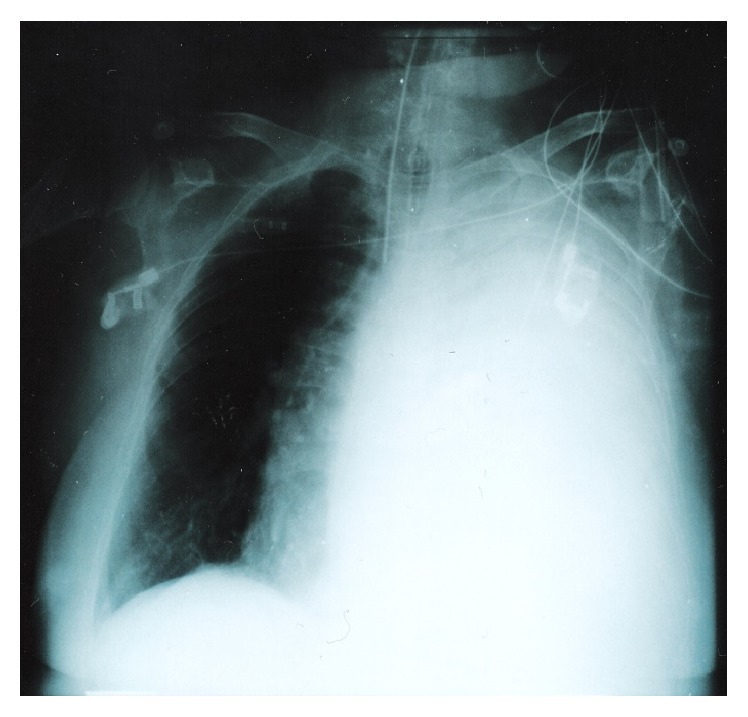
The admission chest X-ray showing complete opacification of the left hemithorax and displacement of the mediastinum to the right.
